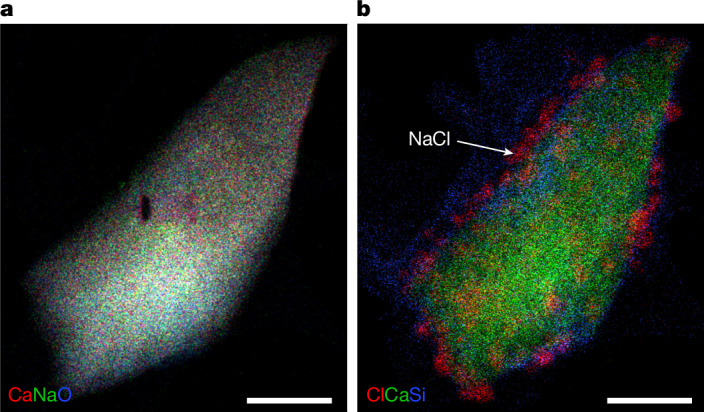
Hydrated Na,Ca carbonate. a, Na,Ca carbonate grain, studied about 1 month following removal from nitrogen storage in the curatorial facility, exhibits SAED *d*-spacing consistent with the hydrated phases gaylussite or pirssonite. The mineral is sensitive to beam damage (dark spot within grain), consistent with a hydrated phase (multielement Kα X-ray map; sample no. OREX-800045-102). b, NaCl particles grown on the same grain after 5 months (multielement Kα X-ray map). Scale bars, 200 nm.
